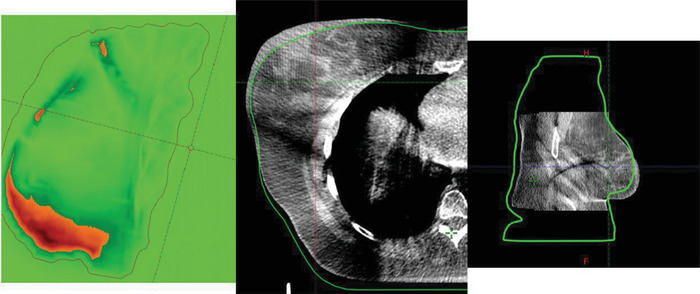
Thirteenth fraction of patient 2. Differences in passing rate are in the lower part of the breast. CBCT images show that the breast is out the external contour in this region, due to an inflammation process
